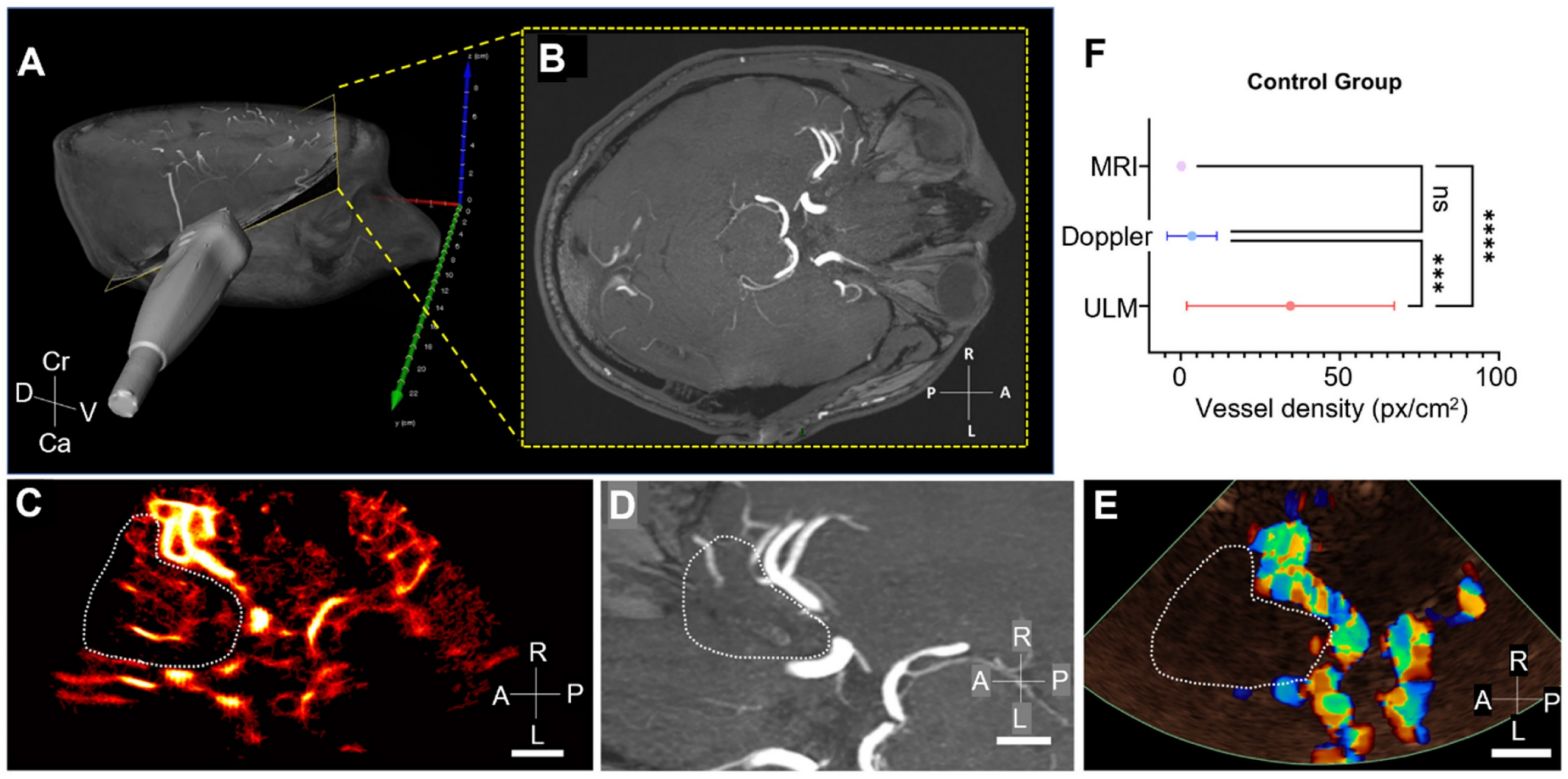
Comparison of vessel density in MRI, ULM, and color Doppler in control patients. A) Position of the phased-array ultrasound probe on the right temporal window in patient 1. Probe is superimposed on a 3D TOF MRI volume in a profile view, B) the yellow-framed slice corresponds to the registered TOF MRI slice in an axial view. C) ULM density map on the first patient (control group). White dotted lines indicate perforating arteries' area segmentation, D) MRI TOF 3T of the same patient registered in b). White dotted lines indicate perforating arteries' area segmentation, E) Color doppler of the same patient. White dotted lines indicate perforating arteries' area segmentation, F) Comparison of vessel density, i.e. number of pixels superior to the median intensity of the image, between the three techniques. One-way ANOVA test (see Methods). Scale bars of 1 cm. Radiological notation, D: Dorsal, V: Ventral, Cr: Cranial, Ca: Caudal, P: Posterior, A: Anterior, R: Right, L: Left.
